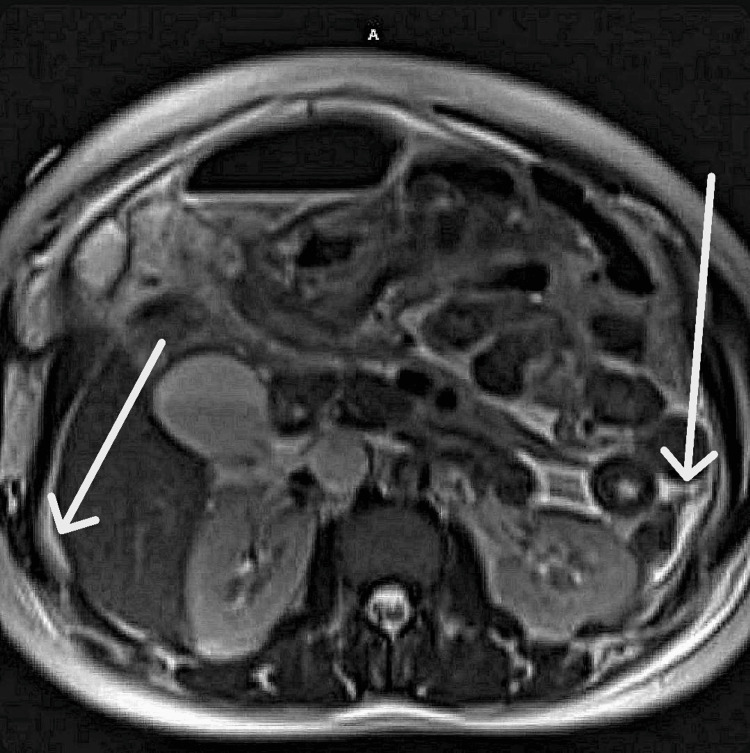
Axial T2-weighted MRI of the abdomen demonstrating moderate ascites. Axial T2-weighted MRI of the abdomen demonstrating moderate-volume ascites. The left arrow indicates perihepatic free fluid, while the right arrow highlights additional intraperitoneal fluid within the abdomen. Gaseous distension of bowel loops is also noted.
